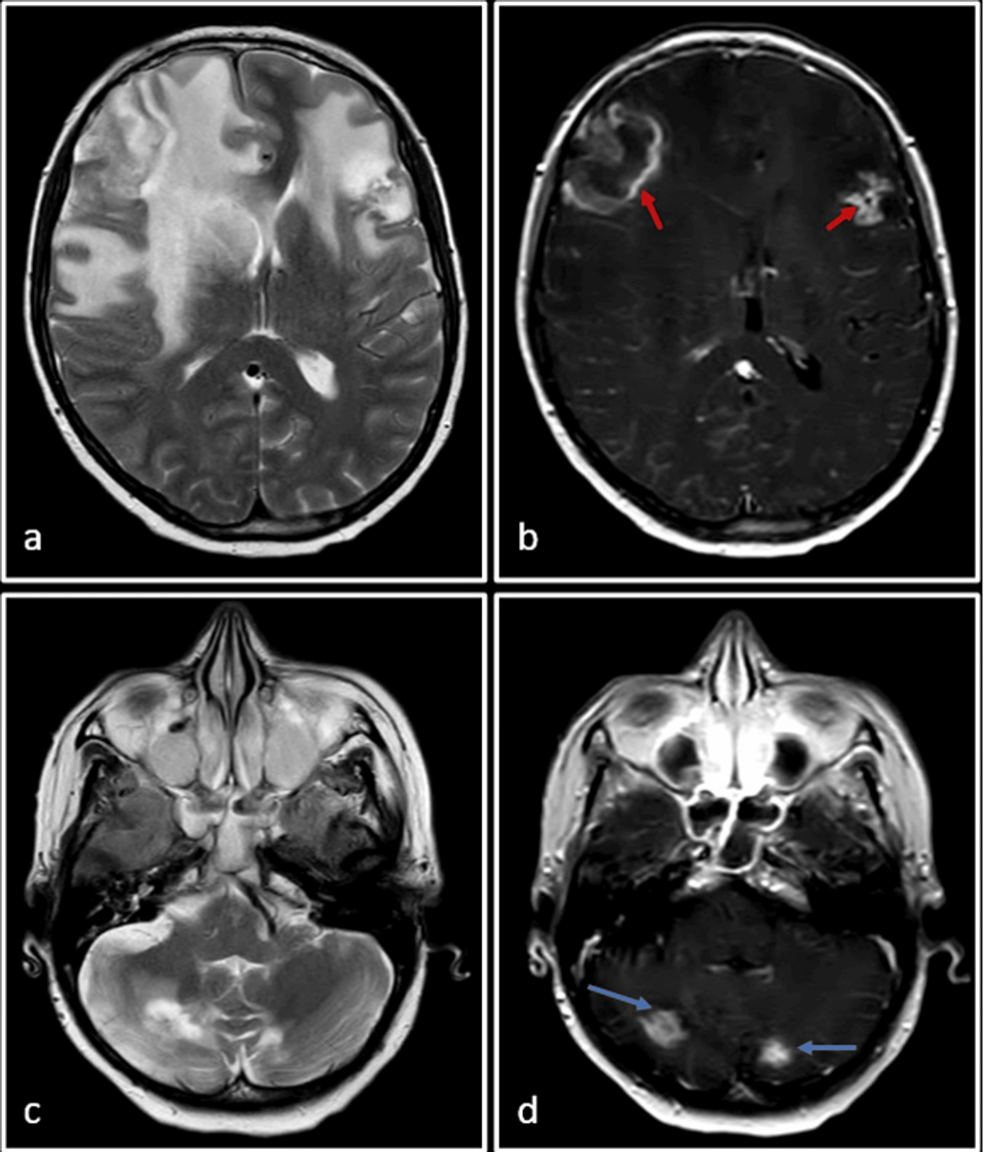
Follow-up MRI after 12 months T2-weighted (a and c) and post-Gadolinium T1-weighted (b and d) images. Note the increase in the size of the right frontal lobe lesion (a and b) with new onset peripheral nodular enhancement (red arrow) in both the frontal lobe lesions, consistent with true progression. The cerebellar lesions are unchanged in size (blue arrows).
